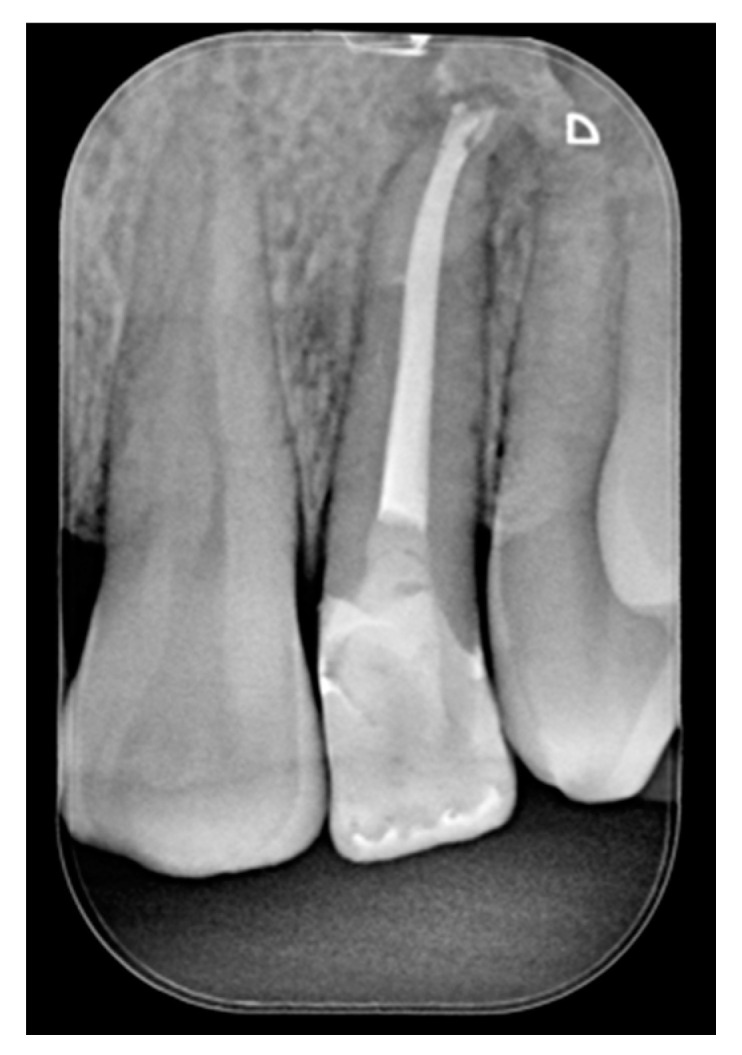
Six-months post-operative x-ray. Reprinted from Restauri diretti nei settori anteriori, G. Paolone, S. Scolavino, © 2021, with permission from Quintessence Publishing Italy.
